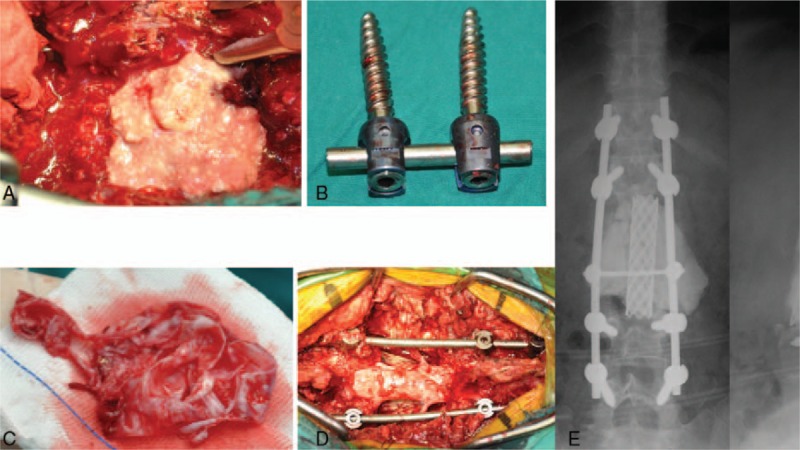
The surgical procedure of our center. (A) The tofukasu-like tissue of the cyst was observed in operation. (B) The former internal fixation screw–rod system was removed. (C) The cyst wall of the mass was removed completely. (D) There was no lesions residual. The screw–rod system reconstructed the spinal structure. (E) The x-rays after the operation showed the reconstruction was solid.
